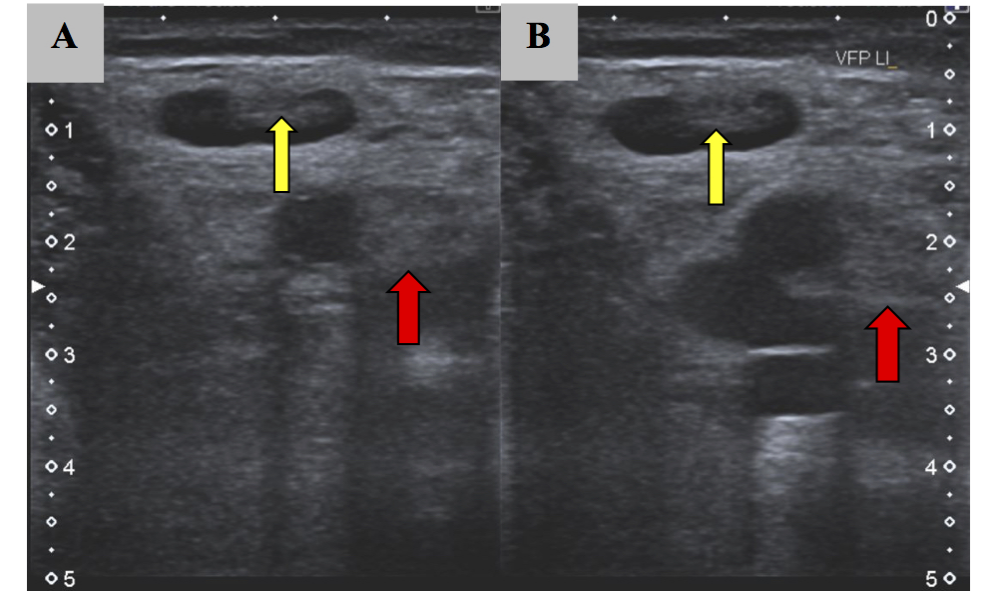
Ultrasound of the left lower extremity showing noncompression of the left saphenous vein (red arrows) and left posterior tibial vein (yellow arrows), as well as a visible thrombus in the lumen. Panel A and Panel B show a well-formed Thrombus in two different locations.
